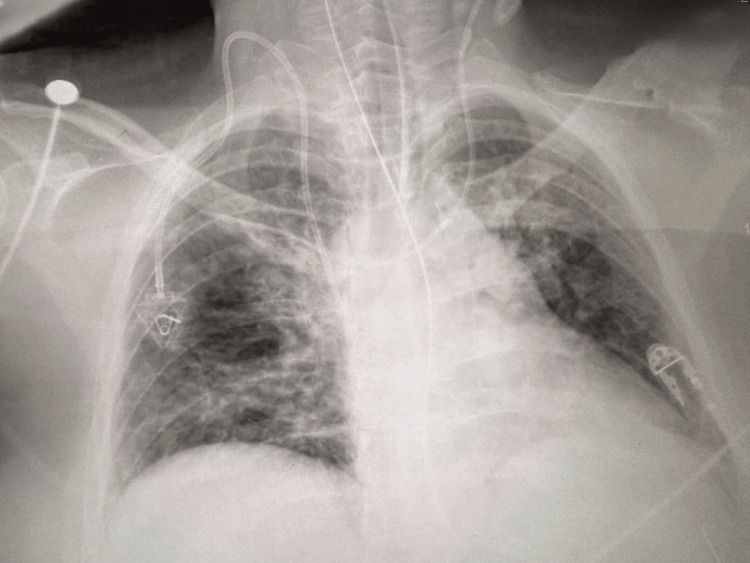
Patient's post-intubation chest X-ray showing mild pulmonary edema
